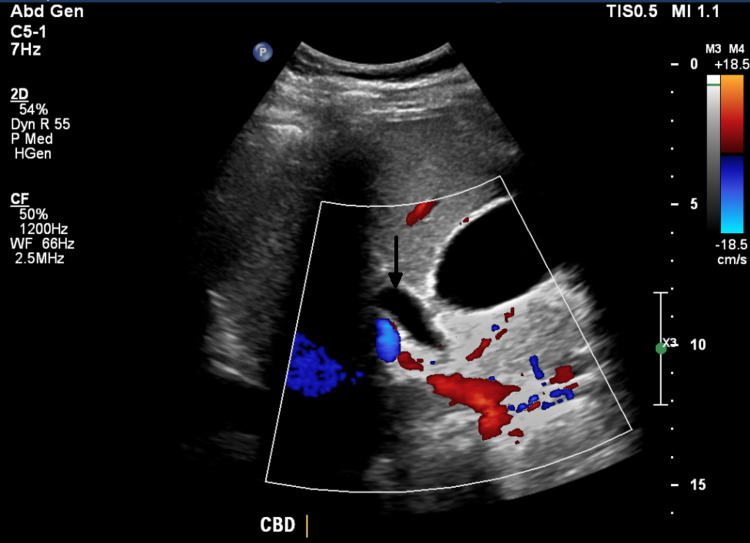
Ultrasound of common bile duct. Dilation of common bile duct to 0.9 cm. Arrow shows dilation of the common bile duct to 0.9 cm as it abuts the gallbladder. CBD: common bile duct.
